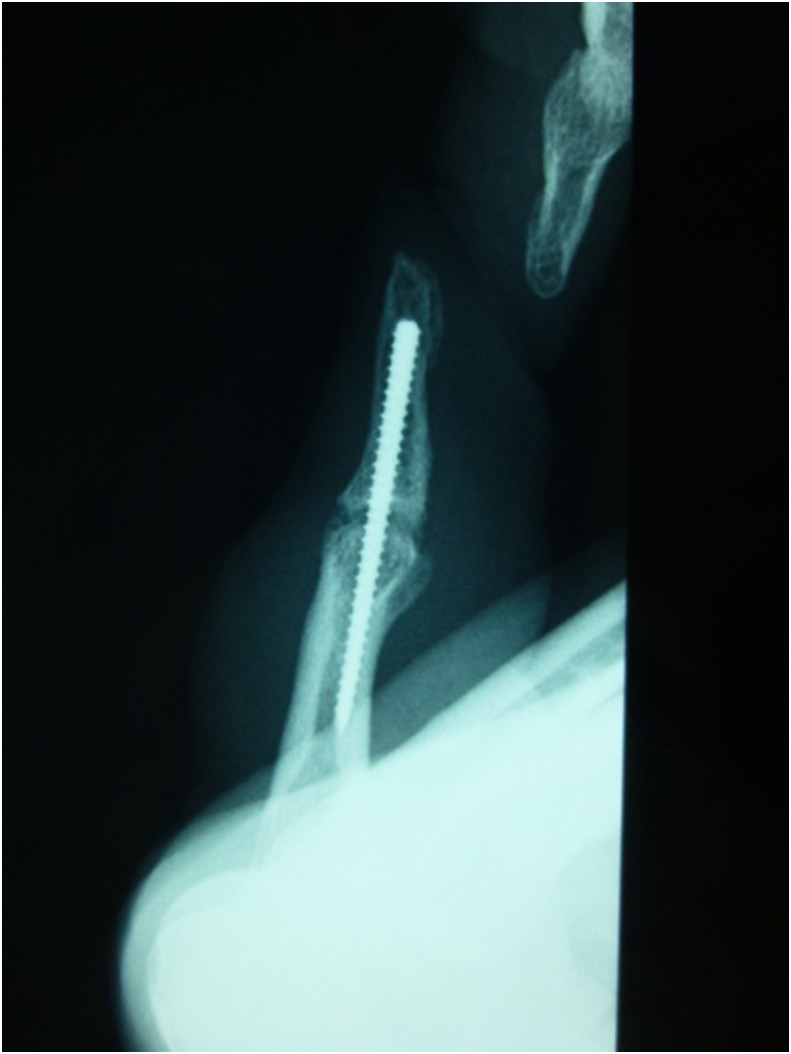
Plain radiography on the final follow-up (2 years after surgery). Bone union of the distal and proximal phalanges was confirmed on plain radiography.
